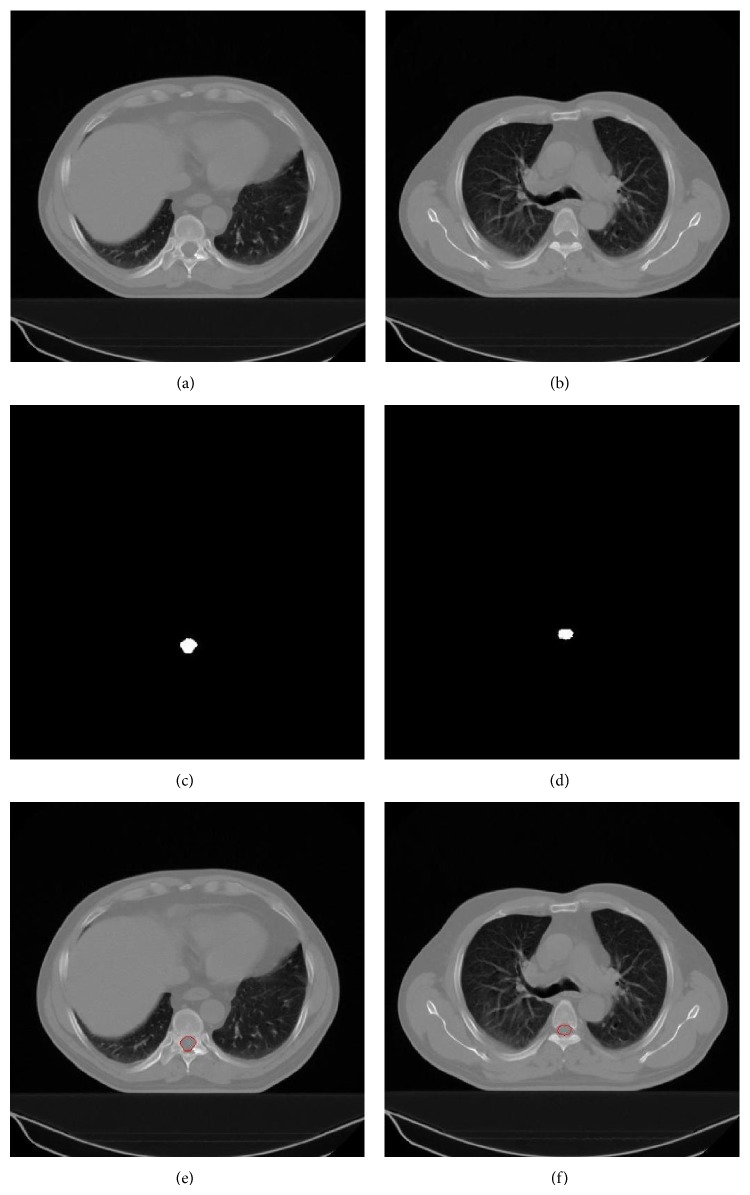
Segmentation of the spinal canal: (a) original CT slice in which the vertebra encloses the spinal canal completely, (b) original CT slice in which the vertebra does not enclose the spinal canal completely, (c, d) segmented spinal canals of (a, b) in white, and (e, f) spinal canal contours in red superimposed on the original slices.
